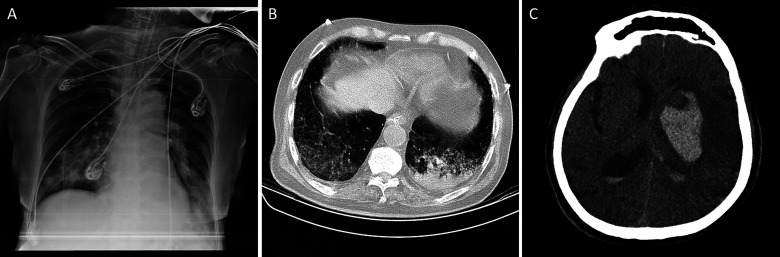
Chest X-ray (a) and chest computer-assisted tomography scan without contrast of chest (b) and brain (c) of a COVID-19 positive patient with severe hemorrhagic stroke.
